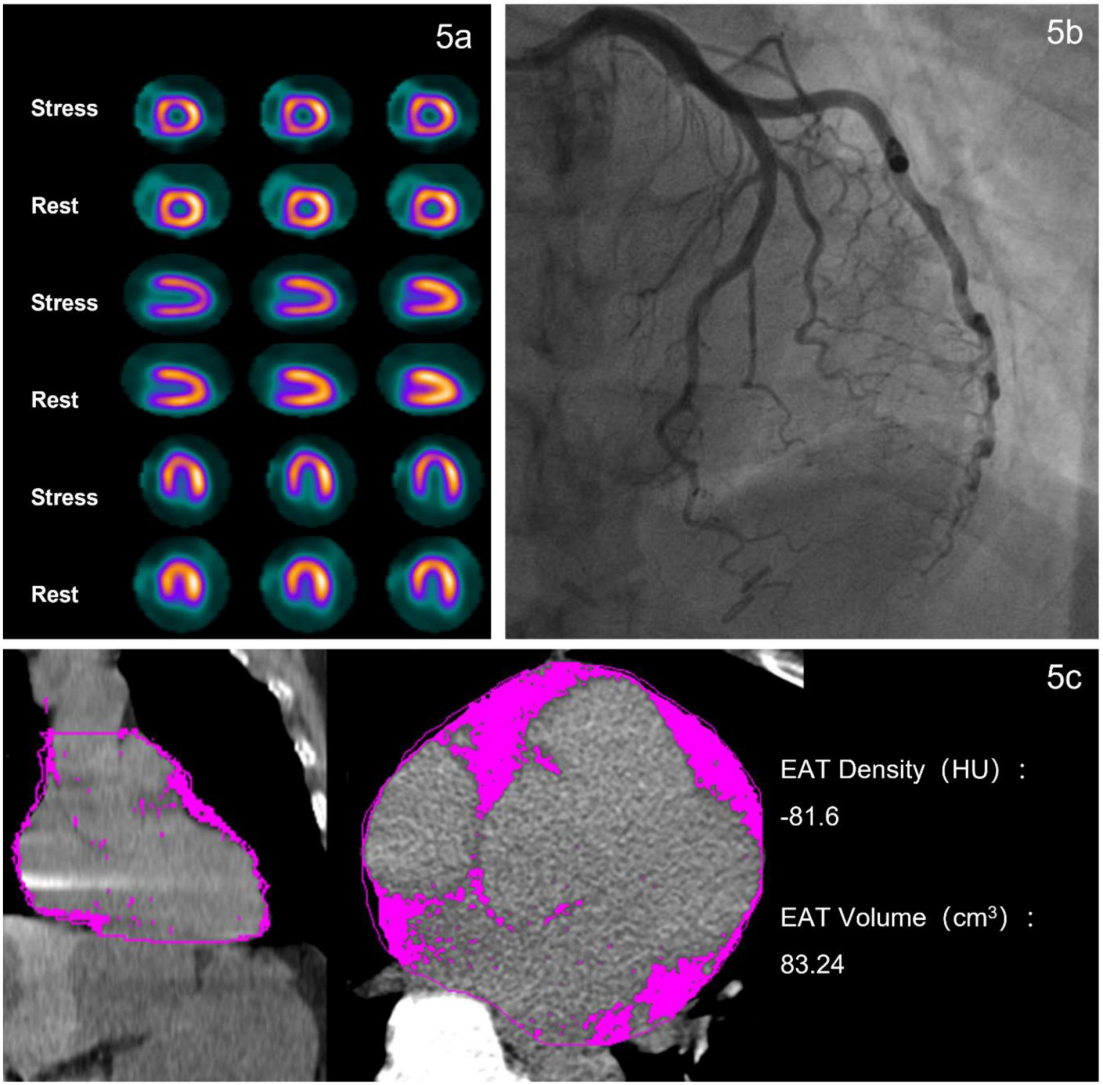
Typical case of patient with no ischemia. A 57-year-old female patient was admitted to the hospital due to chest pain for 2 months. Blood test indicators showed CRP 0.5 mg/L, BNP 31.3 ng/L, and cTnI0.0035 ng/mL. (a) The examination results showed no significant myocardial ischemia on stress-rest myocardial perfusion imaging. (b) CAG revealed 10% stenosis in the proximal segment of RCA, with no significant stenosis in LAD or RCA. (c) Non-contrast CT scan displayed the coronal and axial views of EAT (the pink area), with EAT density of −81.6 HU.
